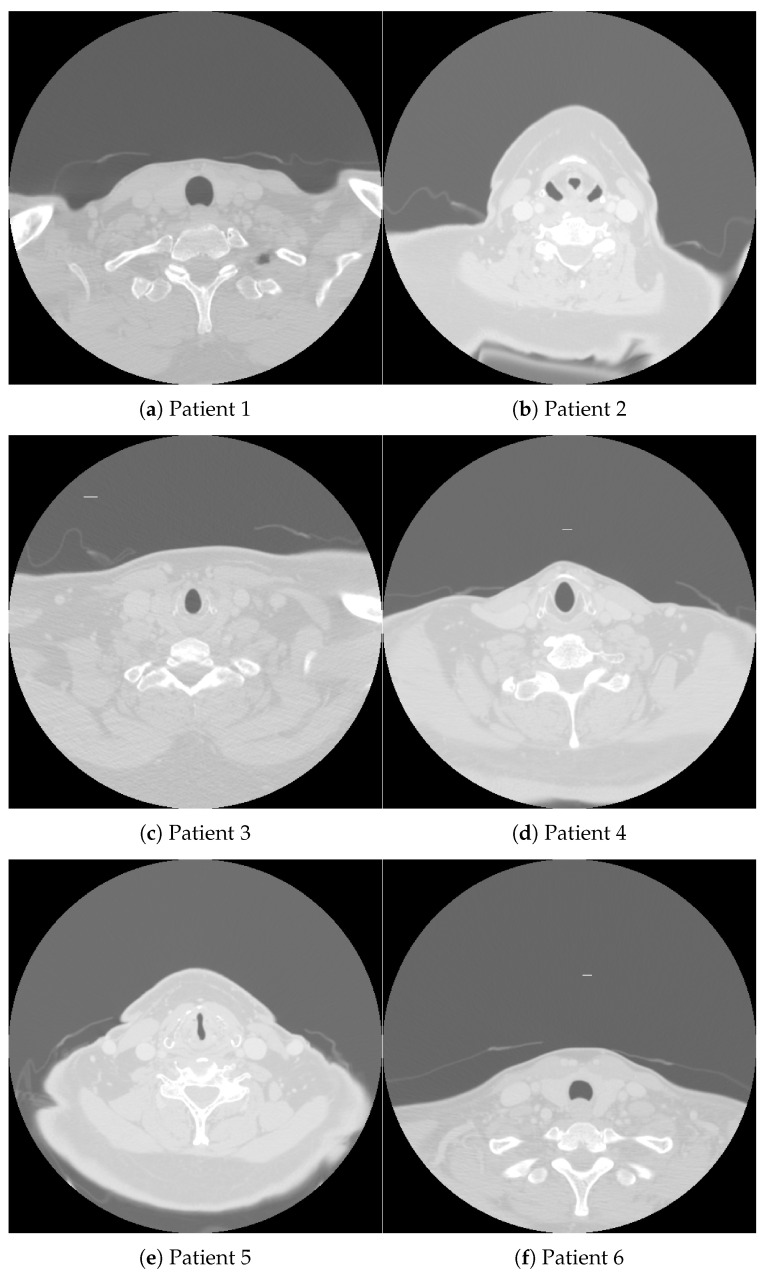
CT images obtained from oropharynx cancer patients whose demographic and clinical data are detailed in Table 1.
